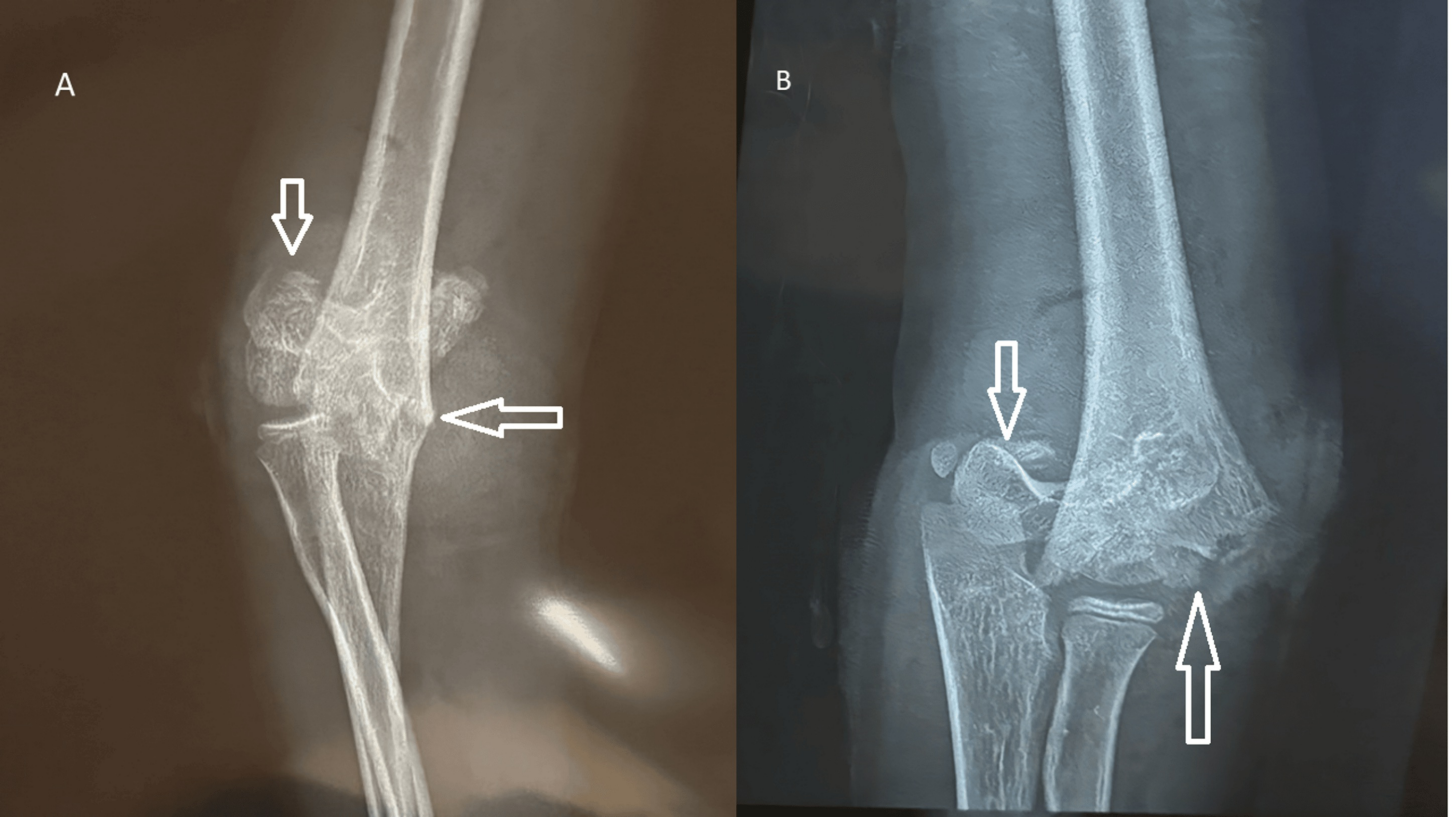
AP (A) and oblique (B) radiographs of the elbow showing a Gartland type III extension-type supracondylar fracture of the humerus A true lateral view could not be obtained due to patient discomfort; a slightly oblique view was acquired instead AP: anteroposterior
